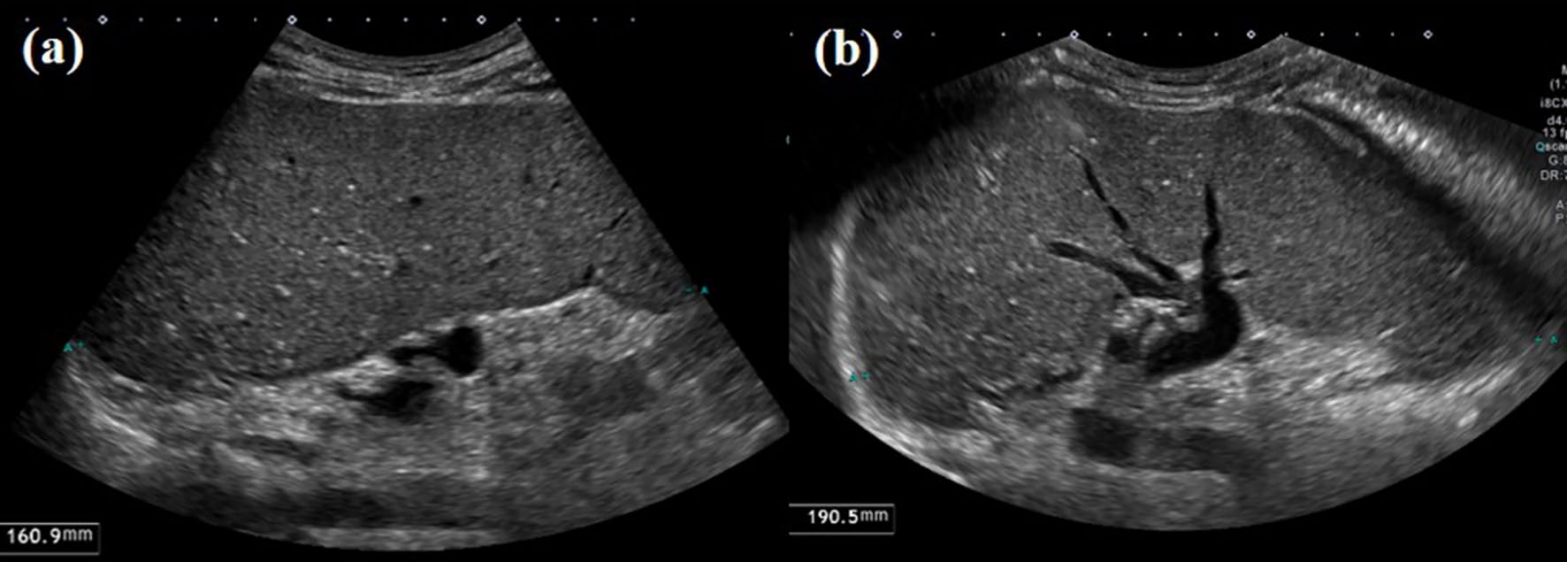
Ultrasound (US) images obtained from a 62-year-old female patient with liver cirrhosis related to hepatitis B viral infection (HBV). a In routine view mode, the limited field of view does not cover the full length of the spleen, resulting in a measured spleen size of 16.1 cm. b Using wide view scan mode, the entire length of the spleen is displayed, resulting in a measured spleen size of 19.1 cm. A CT scan performed six months prior to the US examination measured the spleen size at 18.9 cm
